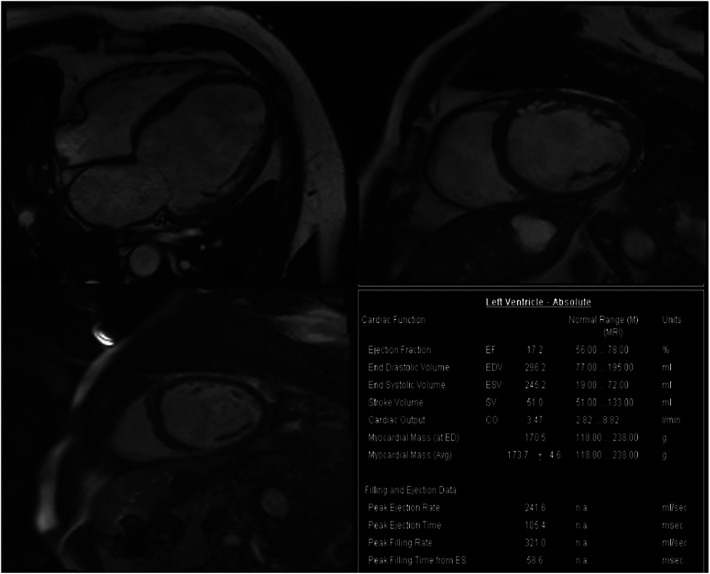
Cardiac magnetic resonance imaging showed DCM. CMR detected dilation of the left ventricle (LVEDV = 296.2 ml), reduction of cardiac systolic function (CO = 3.47l/min, LVEF = 17.2%) and increase of left ventricular mass (LVM = 170.5 g), no signs of storage disease or inflammation. CMR, Cardiac magnetic resonance imaging; LVEDV, left ventricular end-diastolic volume; LVEF, left ventricular ejection fraction; CO, cardiac output.
